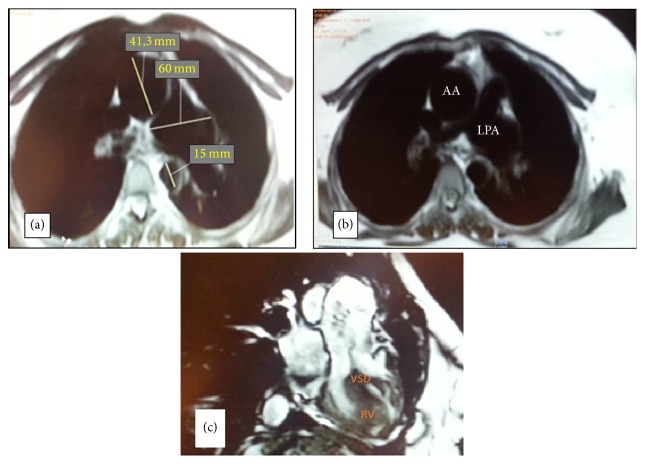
(a and b) The transverse section of a chest MRI showed an aneurysmal change in the left pulmonary artery. Dimensions of the main and right pulmonary arteries were normal. The ascending aorta was also mildly dilated. (c) Cine cardiac magnetic resonance image showing lesions of Fallot's Tetralogy (VSD, overriding aorta, and dilated right ventricle).
